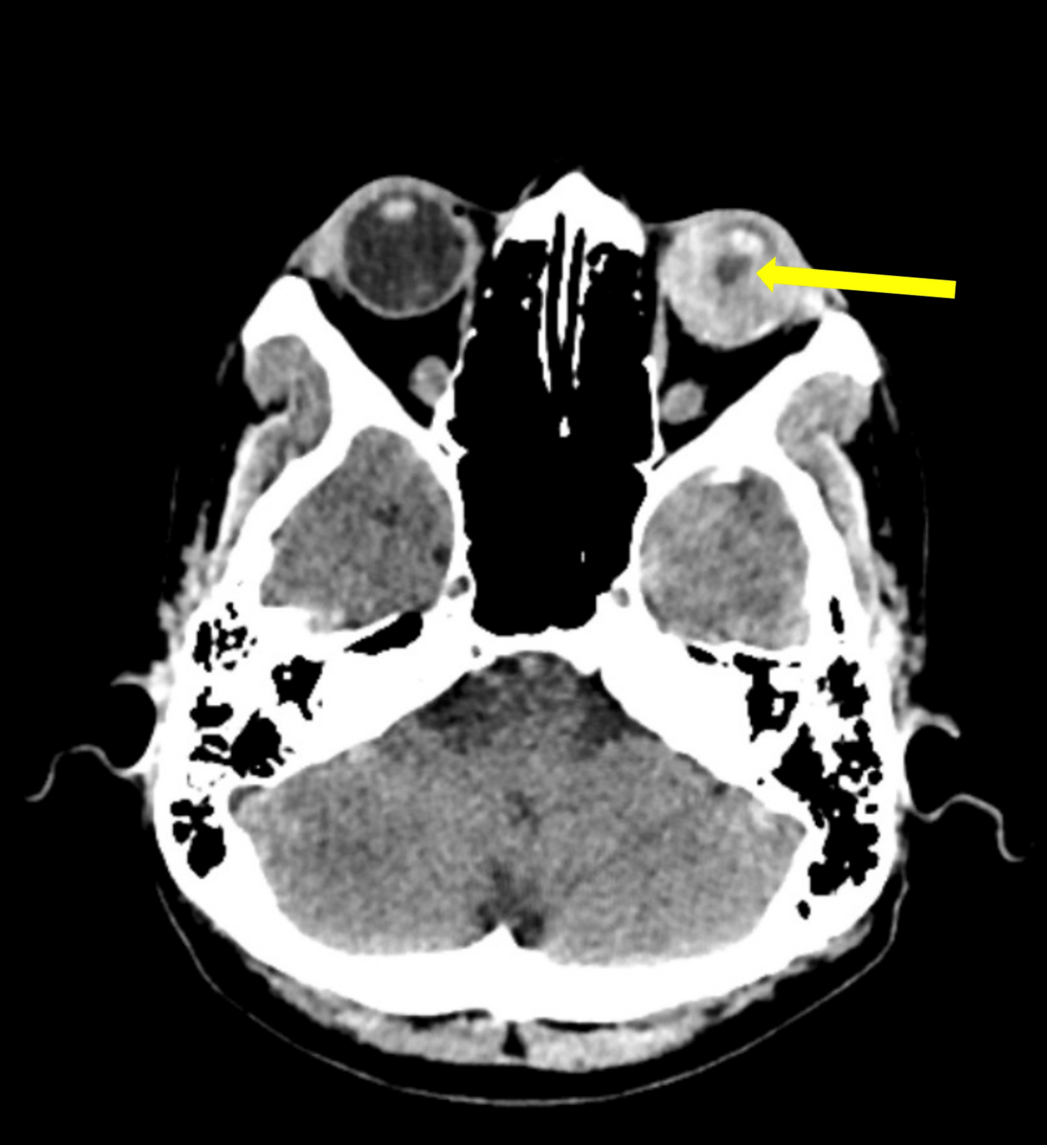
Cranial computed tomography. Yellow arrow indicating hemorrhage in the left eye's posterior chamber.
